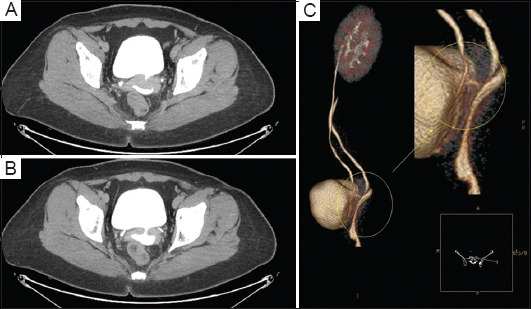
Contrast-enhanced computed tomography images (A and B) in the delayed excretory phase show a full urinary bladder and contrast filling into what was initially believed to be the vaginal vault through a communicating vesicovaginal fistula of around 3 cm in length and of sizable diameter. A digital three-dimensional reconstruction of the excretory phase (C) highlights the fistula.
